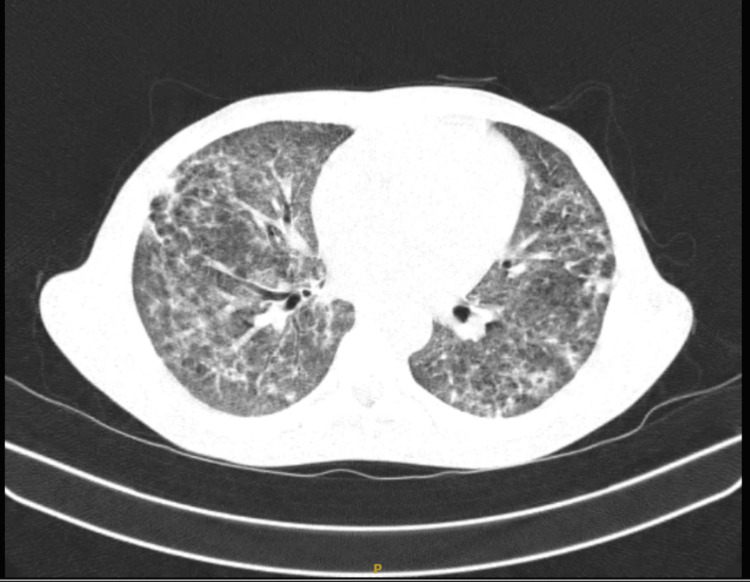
HRCT scan of thorax showing bilateral reticulonodular shadows with tree-in-bud appearance in the bilateral lower lobe HRCT, high-resolution computed tomography
